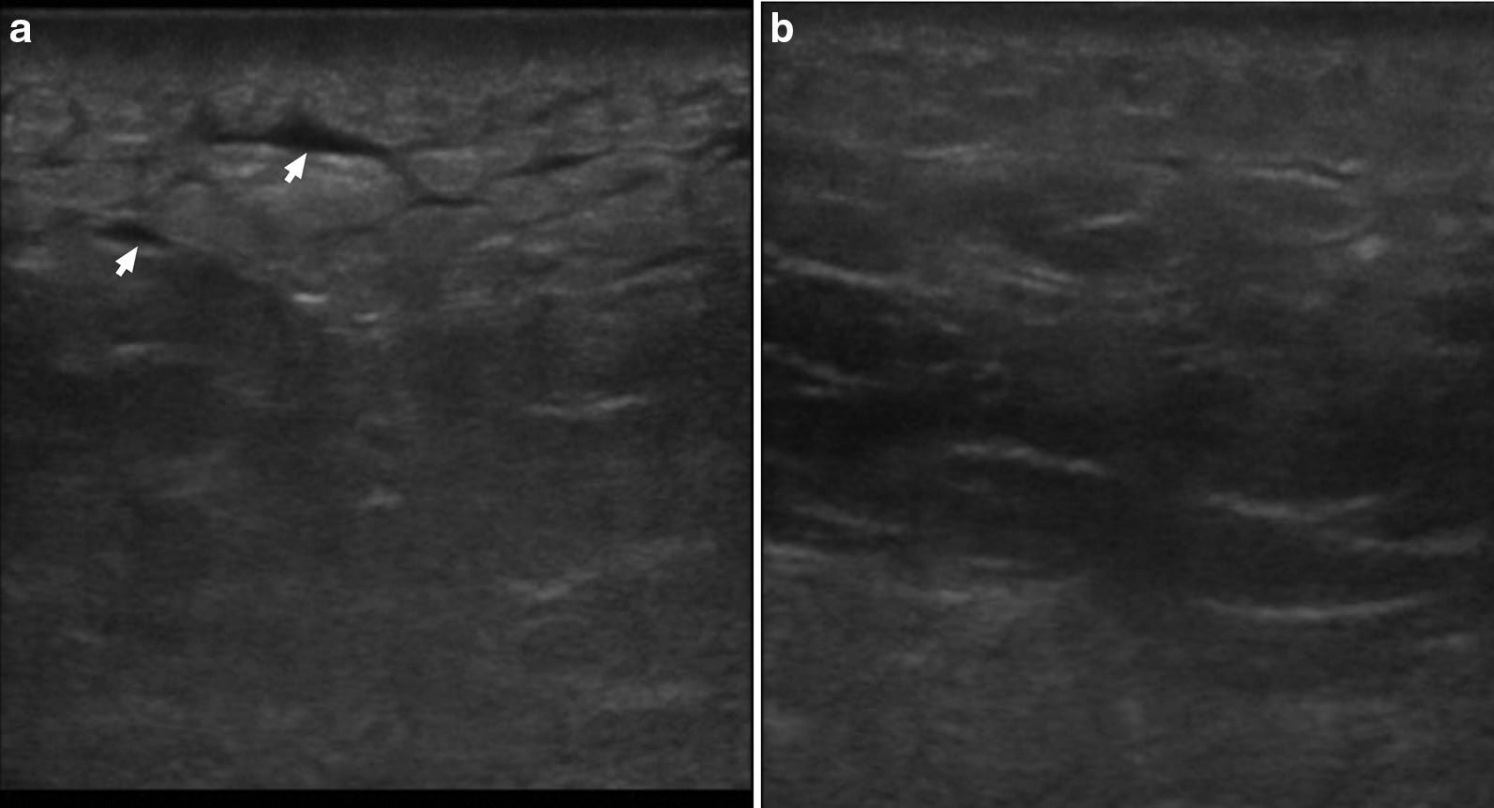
Point-of-care ultrasound scan of the affected left gluteal soft tissue 4 days after admission (a) and 8 days after admission (b) using a 15–6 MHz linear probe. These images show the progression of the sonographic appearance of NSTI, initially with accumulation of fluid in the subcutaneous tissue (arrows), giving it a “cobblestone” appearance and a progressive return to the normal architecture of the subcutaneous tissue over time
